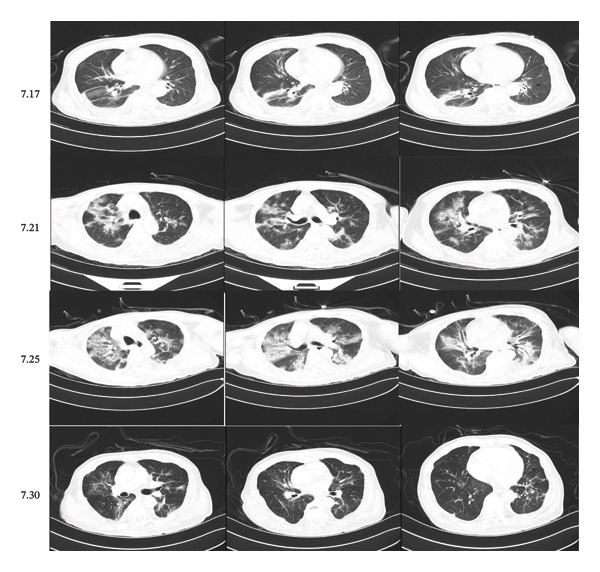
Chest CT changes of patients at different dates.
